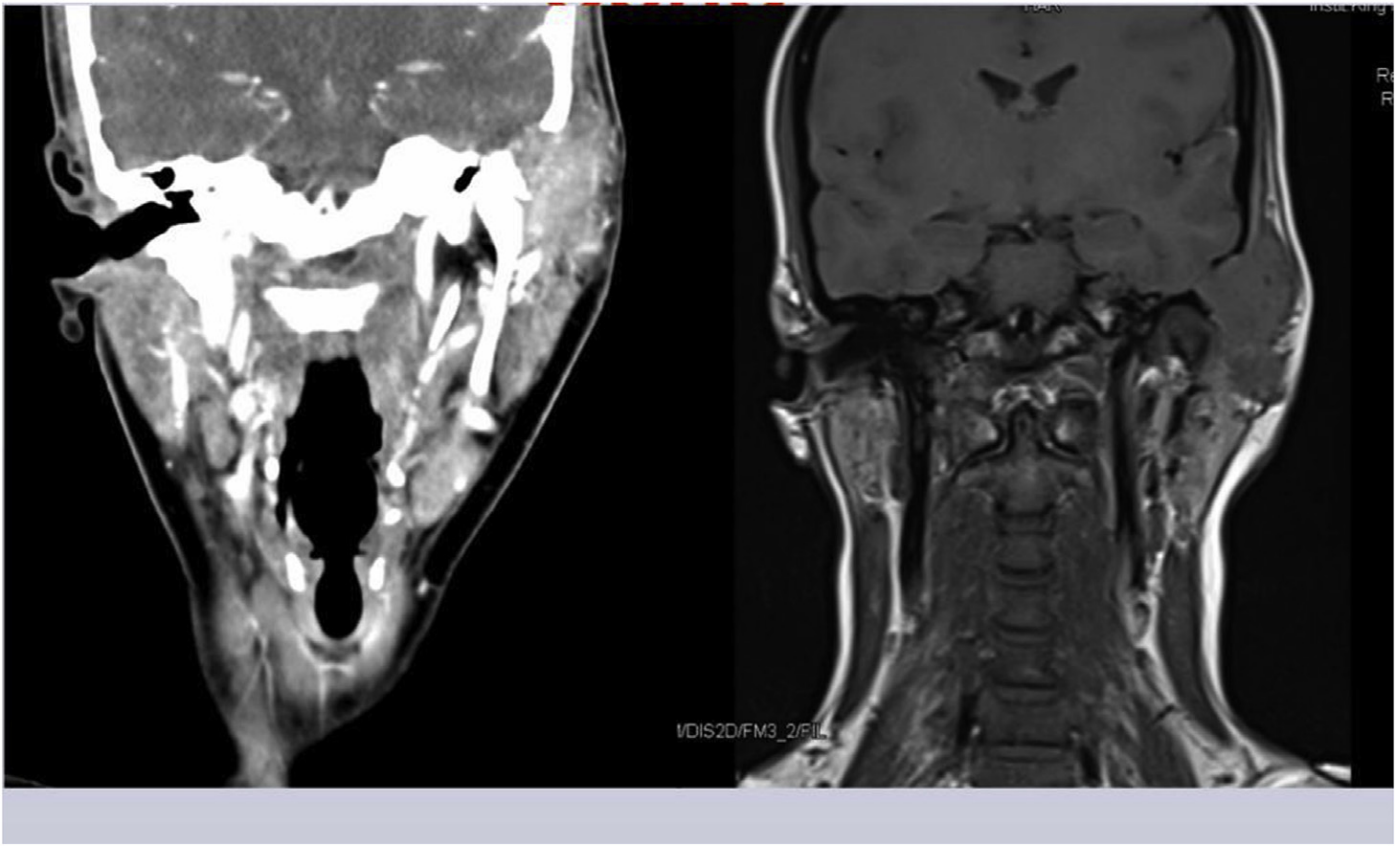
A Contrast-Enhanced Computed Tomography (CECT) displaying the mass with infiltration to the superficial lobe of the left parotid gland. Erosion of the left glenoid fossa, left temporal and zygomatic bones is seen.
